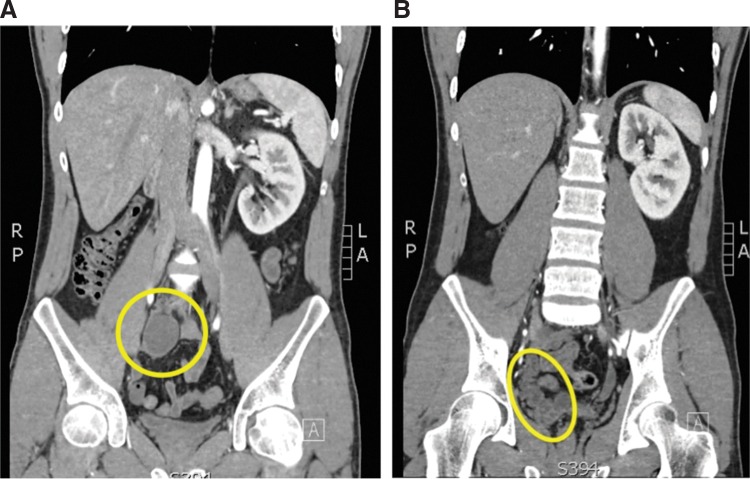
Atrophic and ectopic right kidney. (A) A coronal cut of the CT scan showing the atrophic right pelvic kidney (*yellow circle*). (B) On this coronal cut of the CT scan, the ureter and seminal vesicle complex can be seen (*yellow ellipse*).
